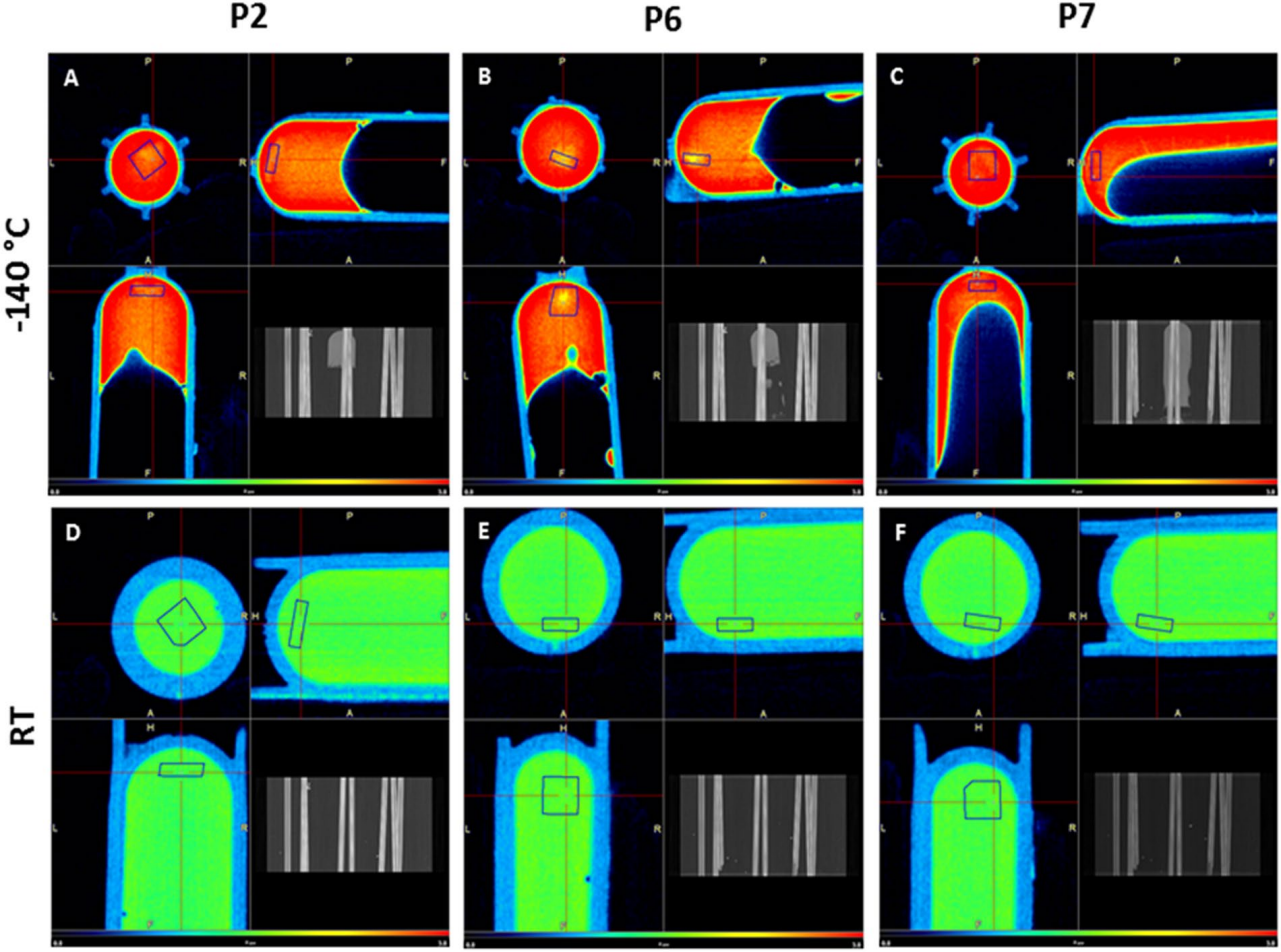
DMSO perfusion assessed by computed tomography. CT images obtained at −140 °C and at RT from three different patients (P2, P6 and P7) showing vitrified and warmed ovarian cortex. Spatial resolution is 0.2 mm and the color scale runs from dark blue for low attenuation (1.2 CT, lower concentrations of DMSO) to intense red for high attenuation (3.0 CT, higher concentrations of DMSO). Blue-colored squares shown in all images are volumes of interest (VOIs) located within the tissue to obtain statistical data on attenuation and DMSO concentrations. Tissue can barely be distinguished from surrounding medium in A and C, while in B it can be partly differentiated, showing less attenuated coloration (yellow) than medium containing 50% DMSO (red). After cryoprotectant removal, there is no difference in attenuation levels in the vials at RT. RT: room temperature.
